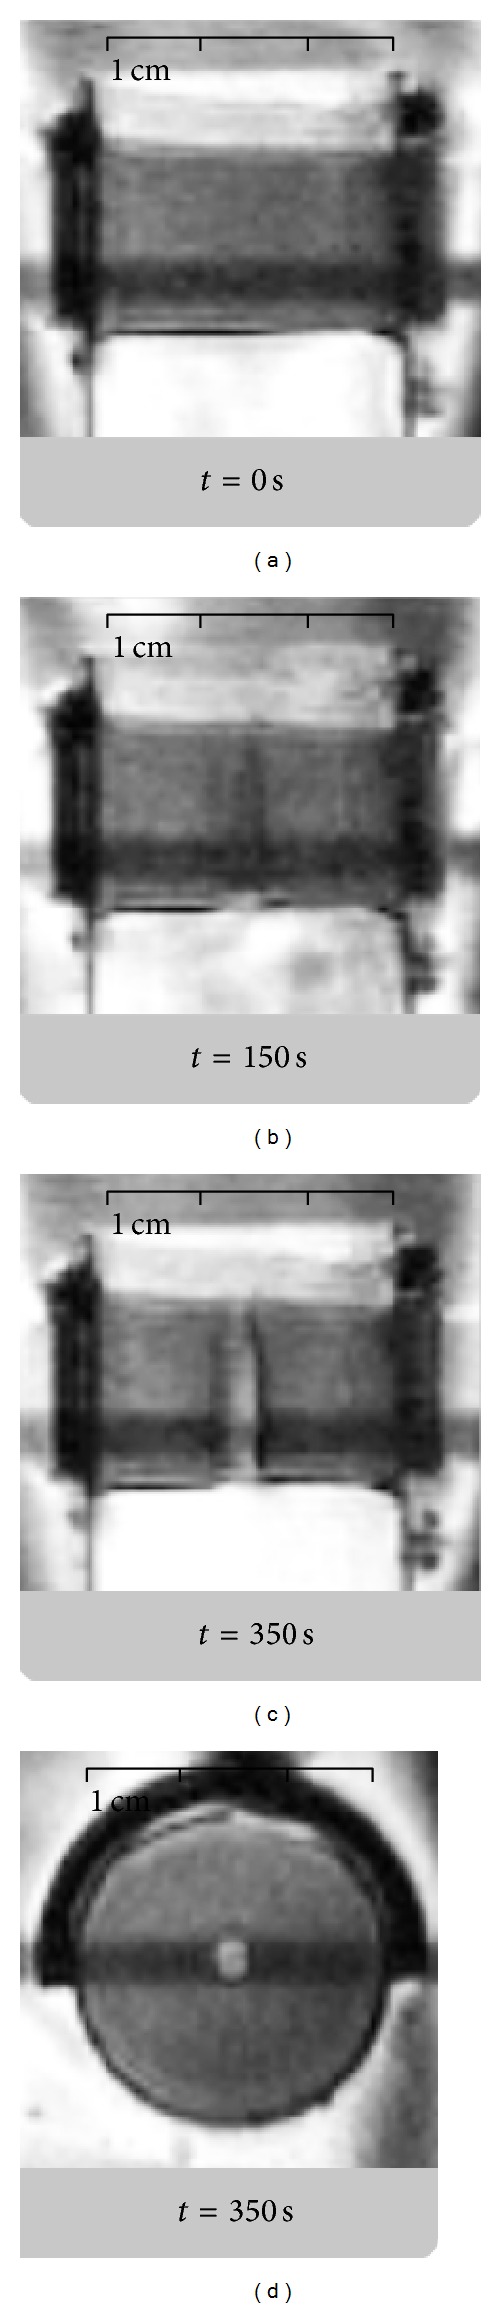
MR imaging of a blood clot during sonolysis (bifrequency excitation, *P*
